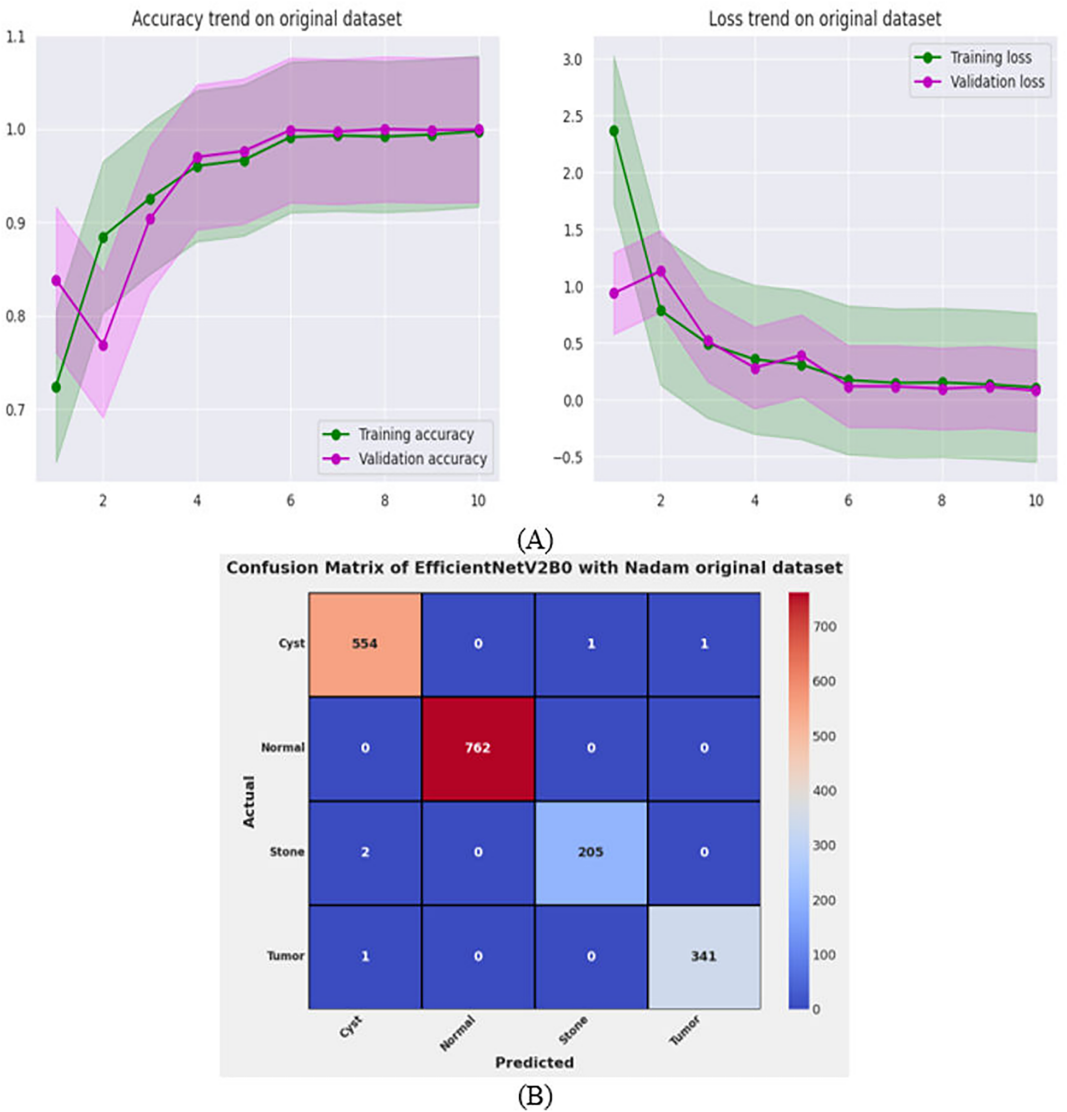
Convergence behavior & confusion matrix of the proposed fine-tuned Efficientnetv2b0 on original CT-kidney test dataset.
